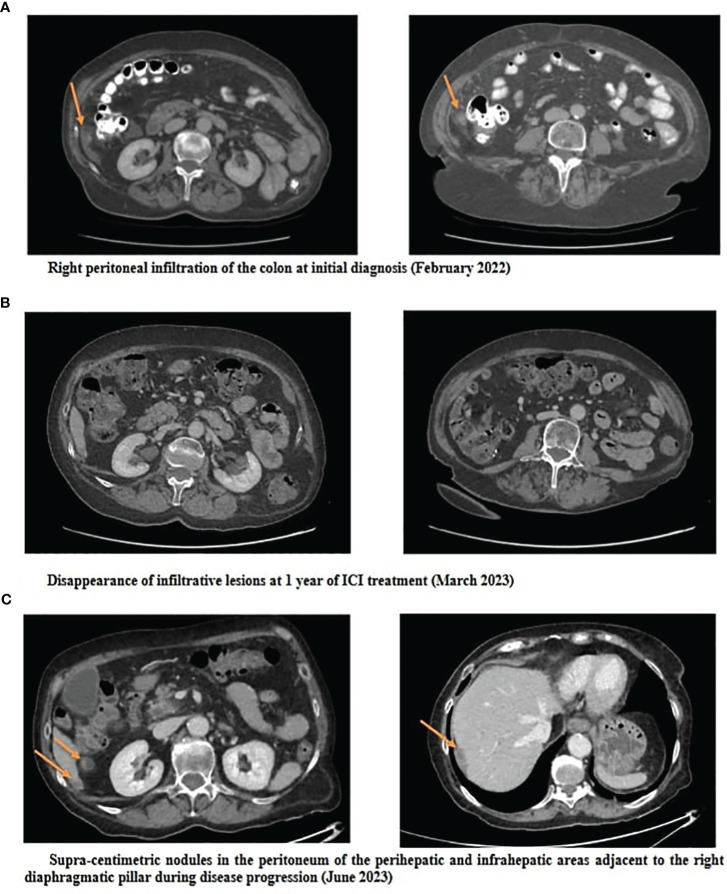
Abdominopelvic CT scans at initial diagnosis (A), at 1 year of ICI treatment (B) and at progression (C).
